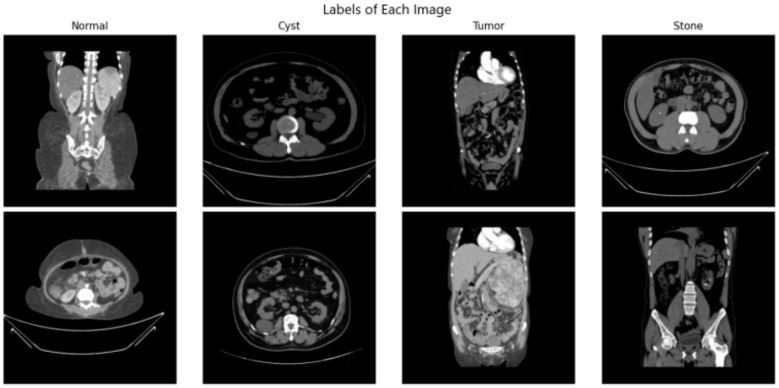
Representative axial and coronal CT slices from the four diagnostic categories in the Islam (15) dataset: (Source: https://www.kaggle.com/datasets/nazmul0087/ct-kidney-dataset-normal-cyst-tumor-and-stone; Creative Commons Attribution License (CC BY): Normal (column 1), Cyst (column 2), Tumor (column 3), and Stone (column 4). All images are sourced from the publicly released, fully de-identified dataset. Top row: axial plane; bottom row: coronal plane. Visual differences in parenchymal texture, lesion morphology, and calcification appearance motivate the four-class taxonomy.
